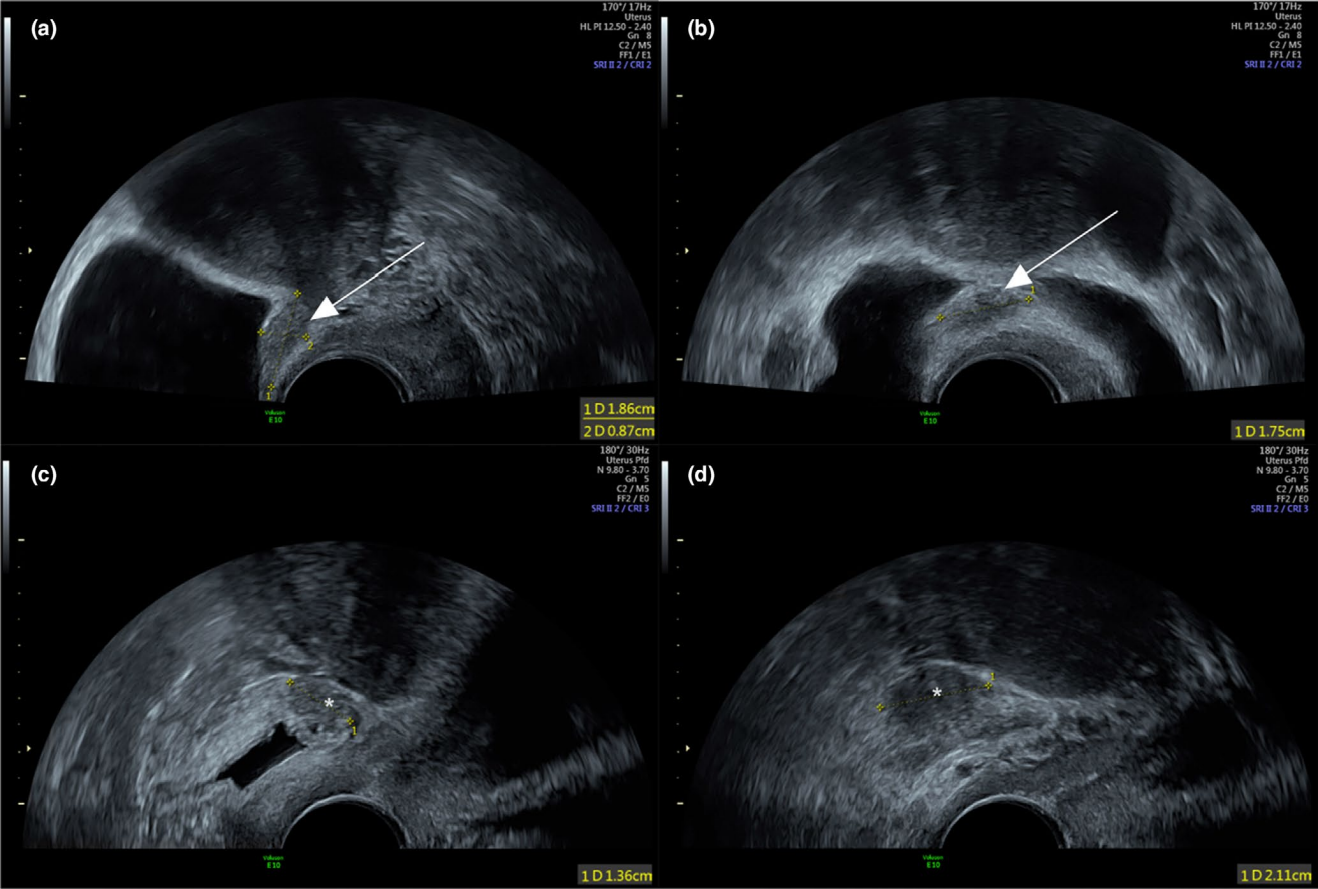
Ultrasound appearance of anterior subperitoneal endometriosis in the vesicouterine pouch. Sagittal (a) and axial (b) endovaginal ultrasound slices showing a 19 × 18 × 9‐mm nodule, isoechoic to the vesicouterine pouch in a 30‐year‐old patient (white arrow). Ultrasound appearance of anterior bladder subperitoneal endometriosis. Sagittal (c) and axial (d) endovaginal ultrasound slices showing a 21 × 14‐mm iso/hypoechoic nodule infiltrating the bladder muscle in a 38‐year‐old patient (white star).
